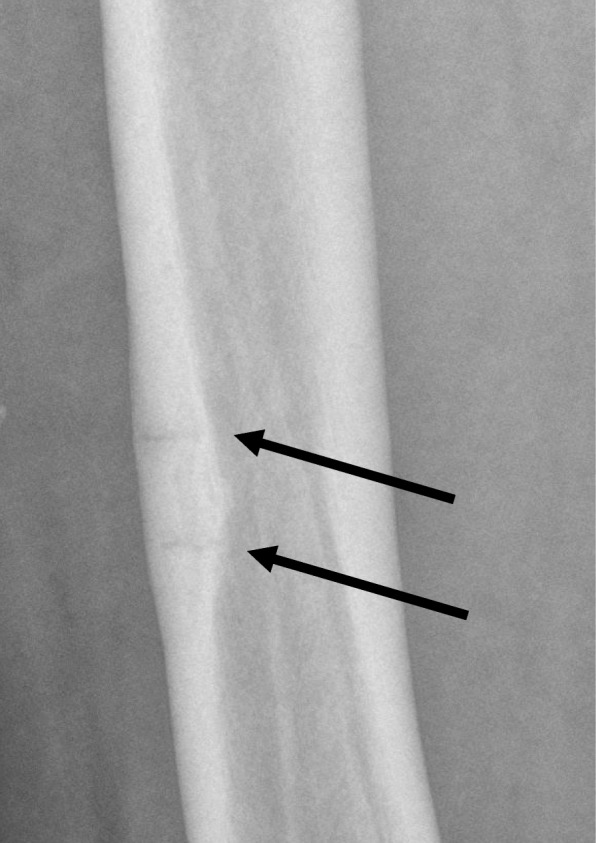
Bisphosphonate insufficiency fractures seen on a plain film of the femur
